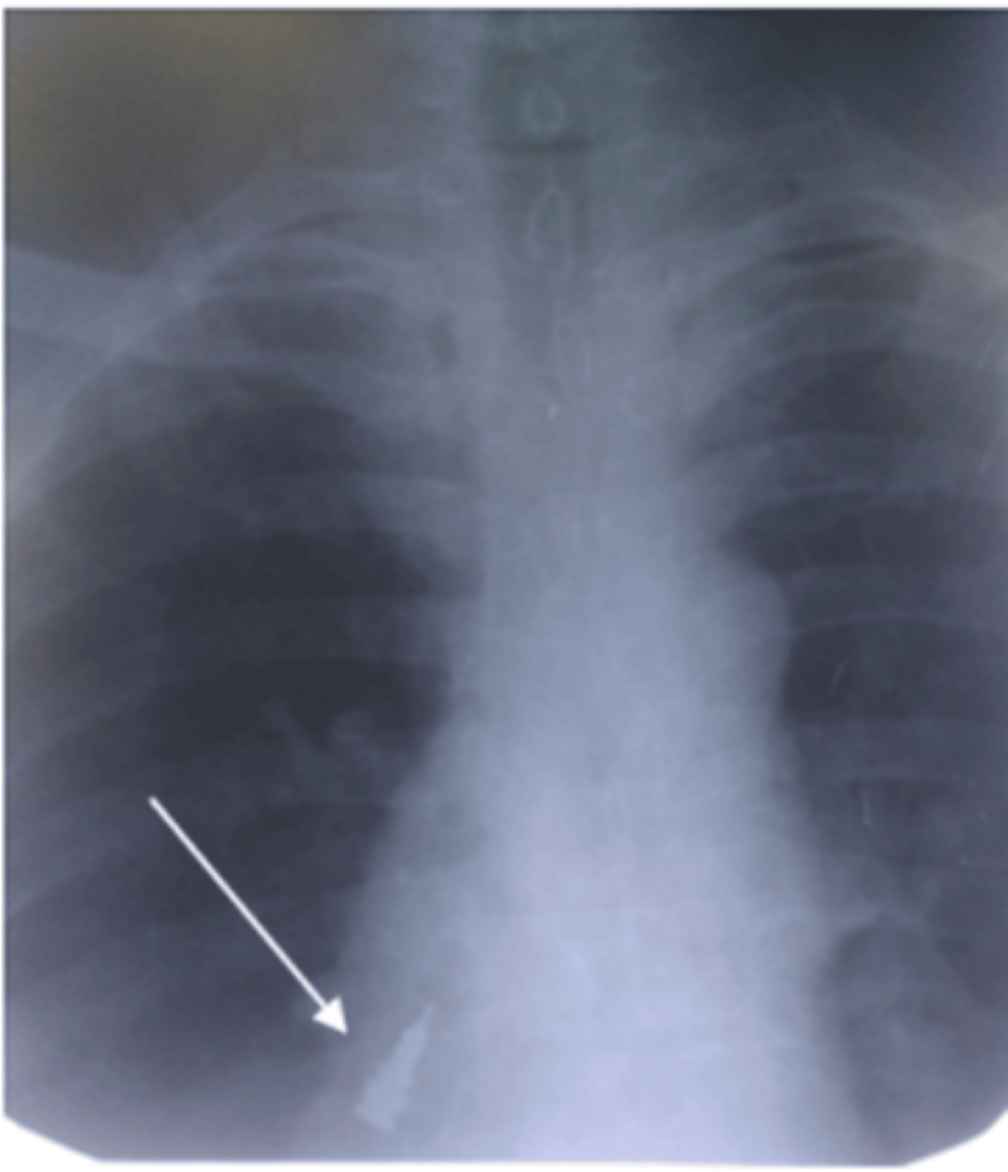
Thoracic and spine radiograph indicating a radiopaque foreign object.
